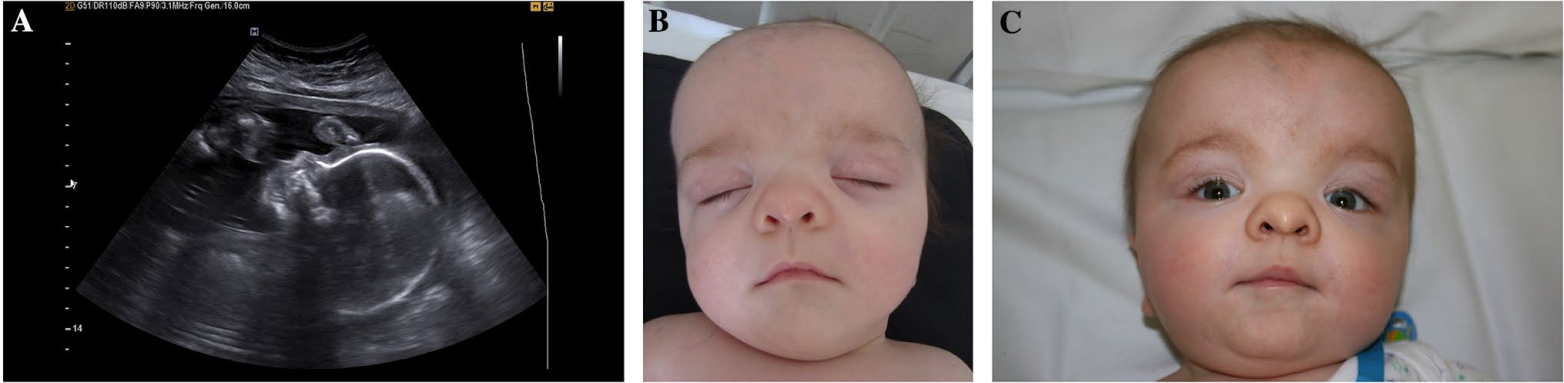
Sonographic and postnatal images of a child with Greig cephalopolysyndactyly syndrome. The cranial biometric parameters were in the normal range, a dysgenesis of the corpus callosum was suspected. After the birth, a high forehead with down-slanting palpebral fissures and a low nose root is seen
